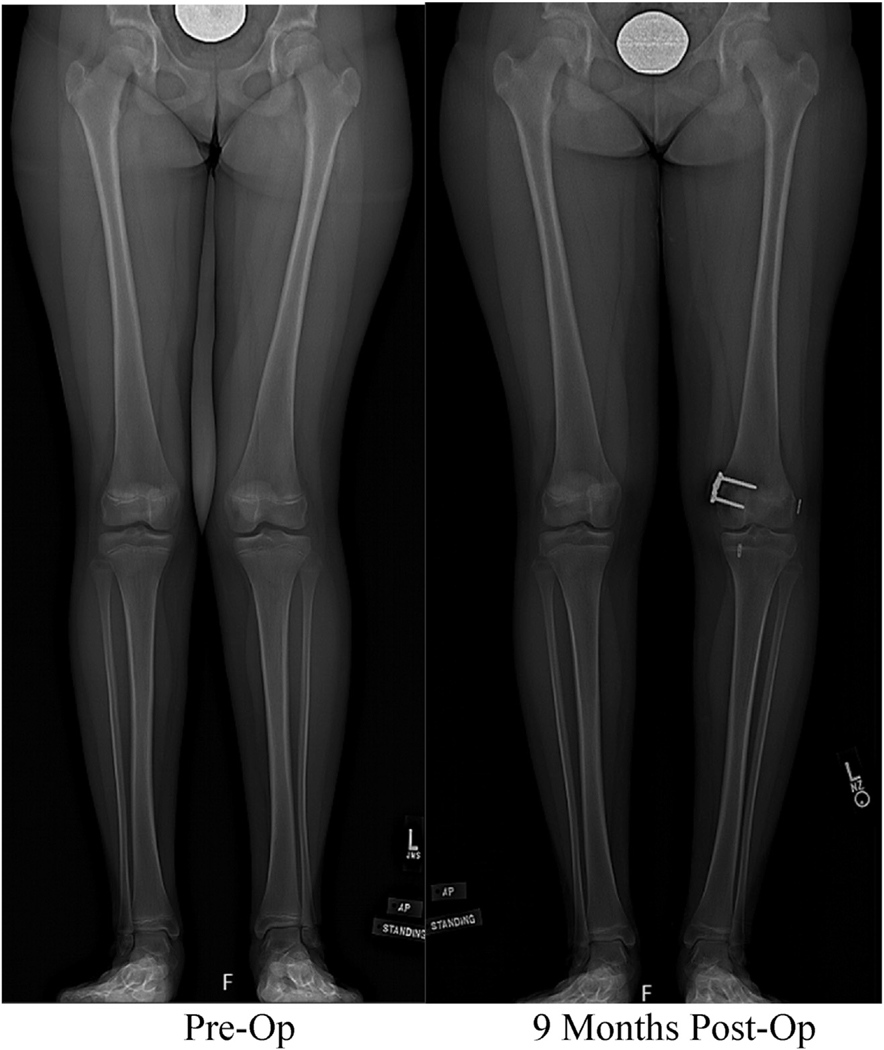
Pre-operative and post-operative full-length lower extremity standing radiographs of a 11-year-old female.
